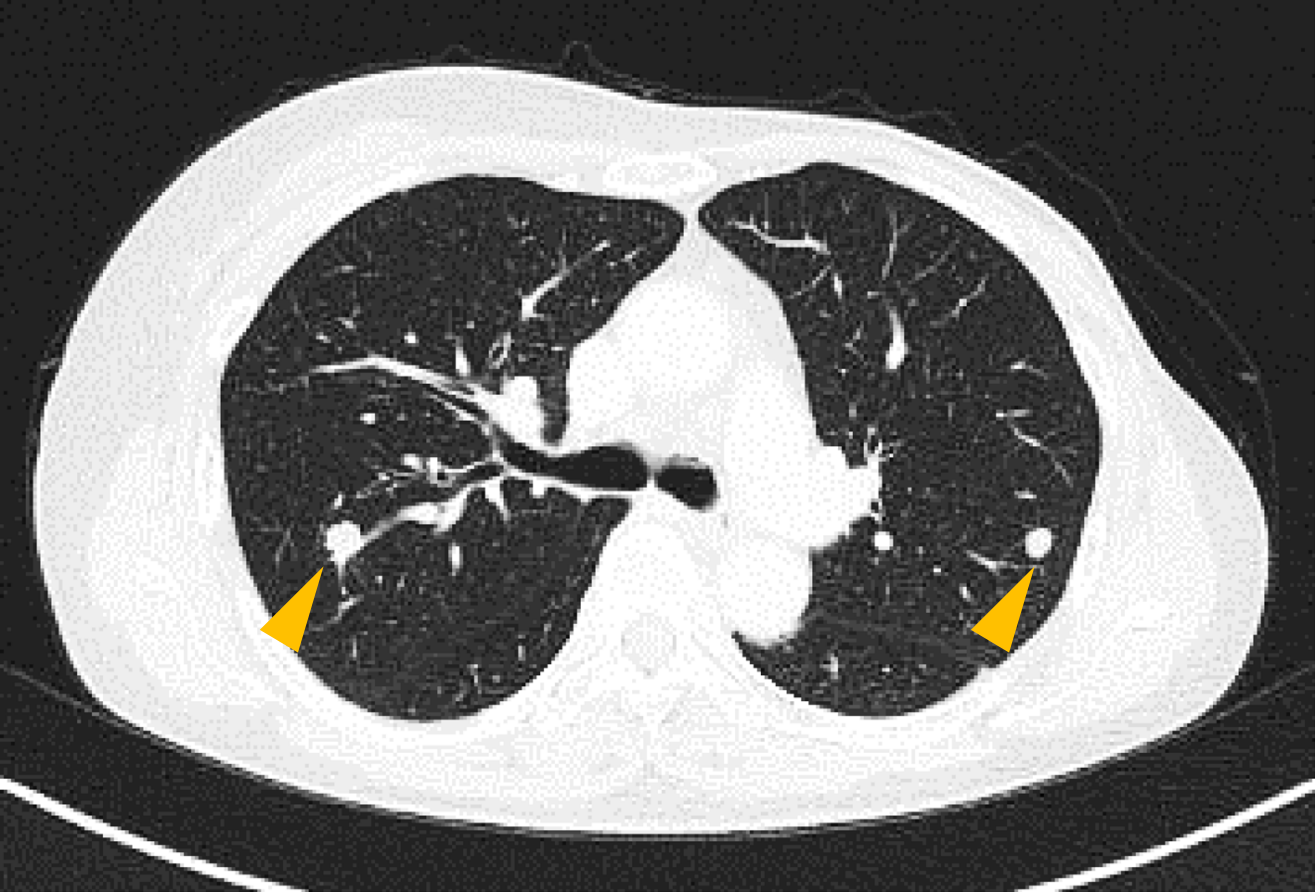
Images of computed tomography (CT) at diagnosis showing lung metastasis. CT revealed two nodular masses located in the patient’s left upper and right upper lobes of the lungs (orange arrowheads)
